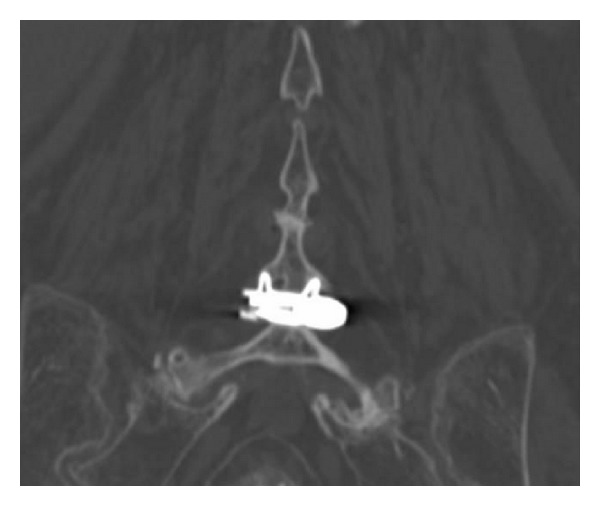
Coronal CT image of the Aperius inserted at L4-L5 level.
